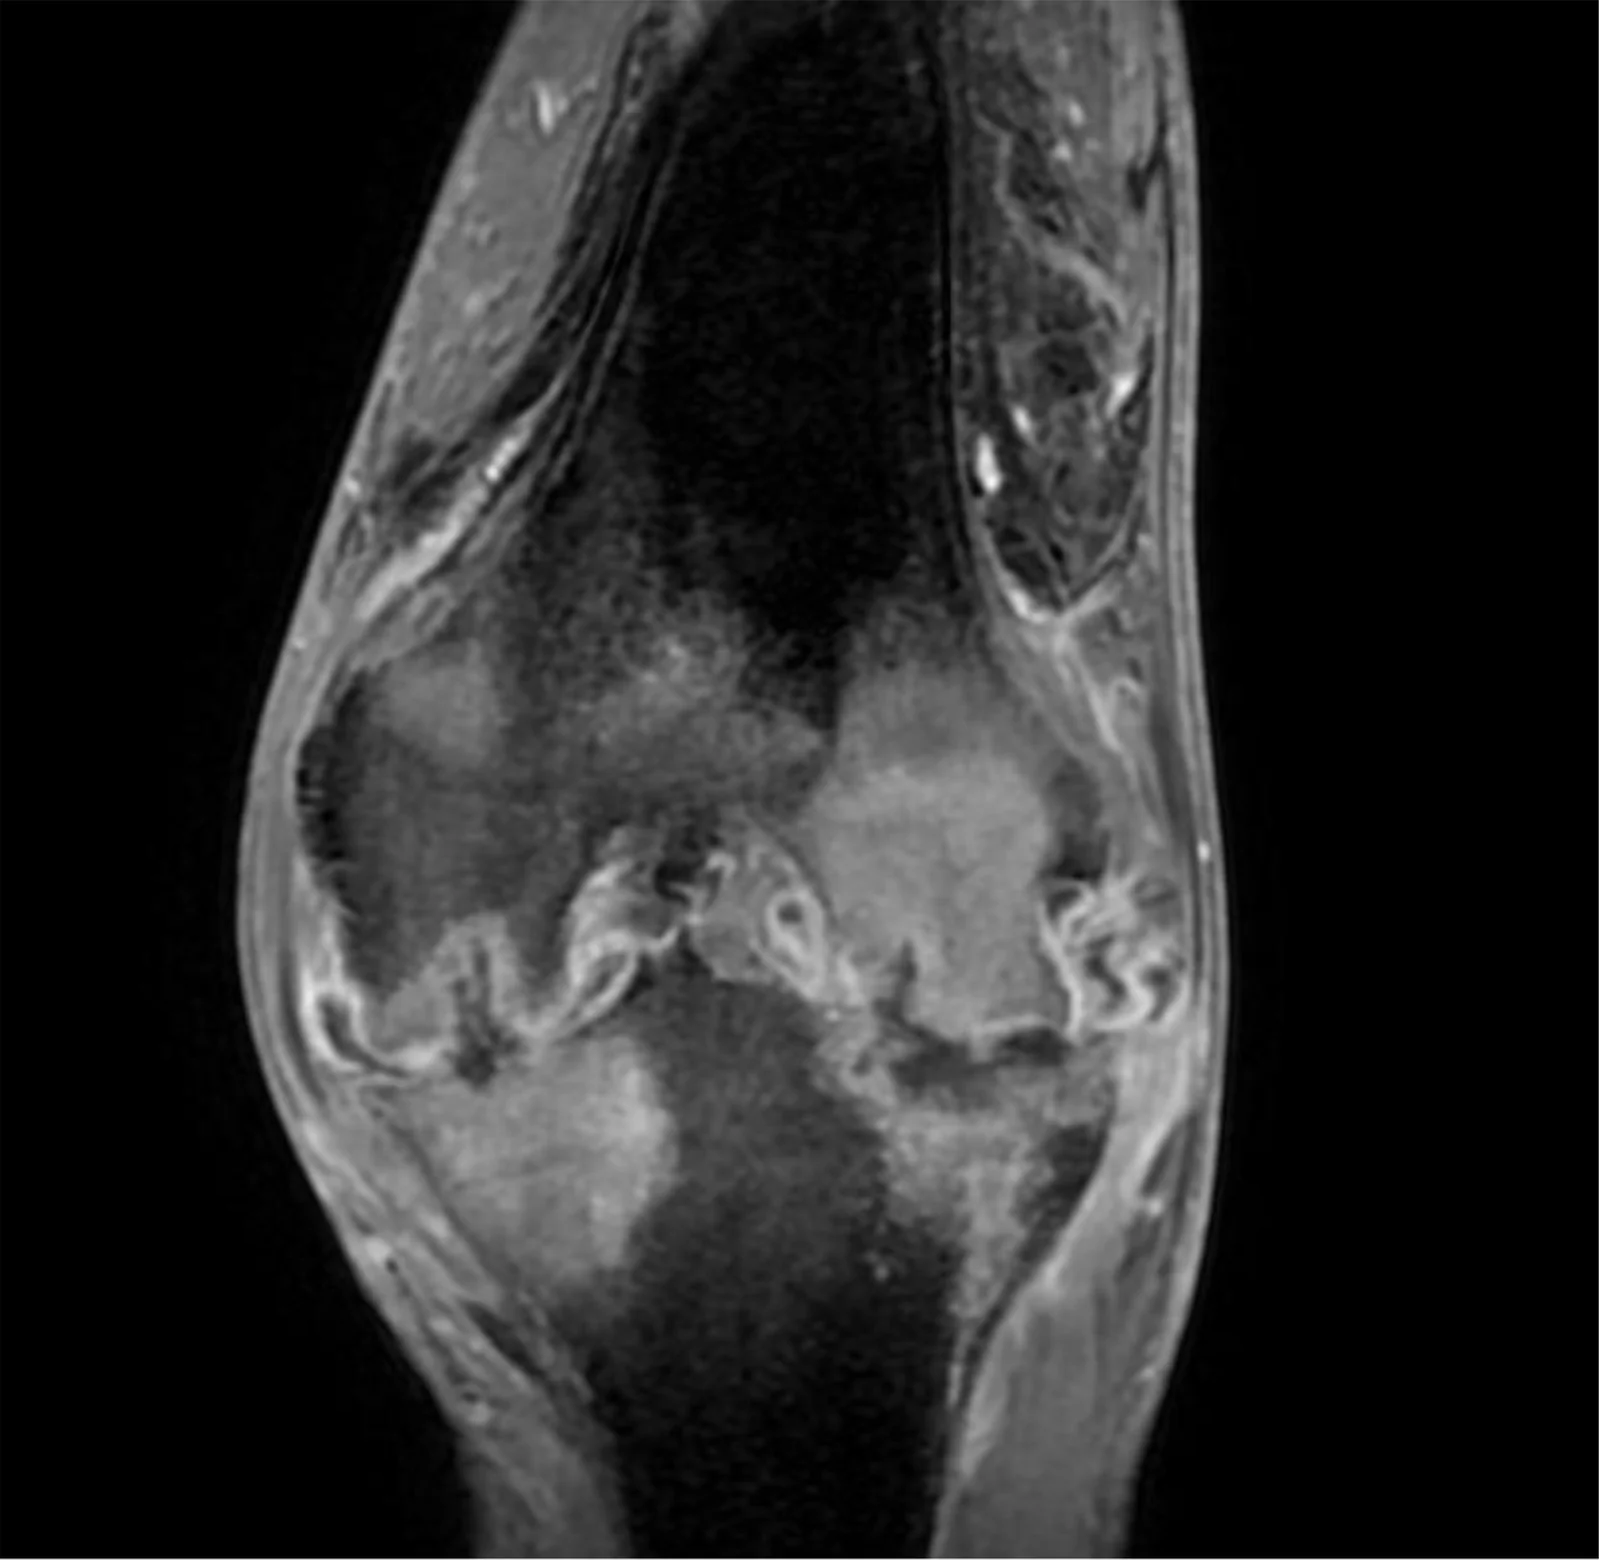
Coronal, post-contrast T1-weighted MRI of the left knee showing joint space narrowing with bone erosion of the tibial and femoral articular surfaces, marked bone marrow oedema and synovial enhancement.
